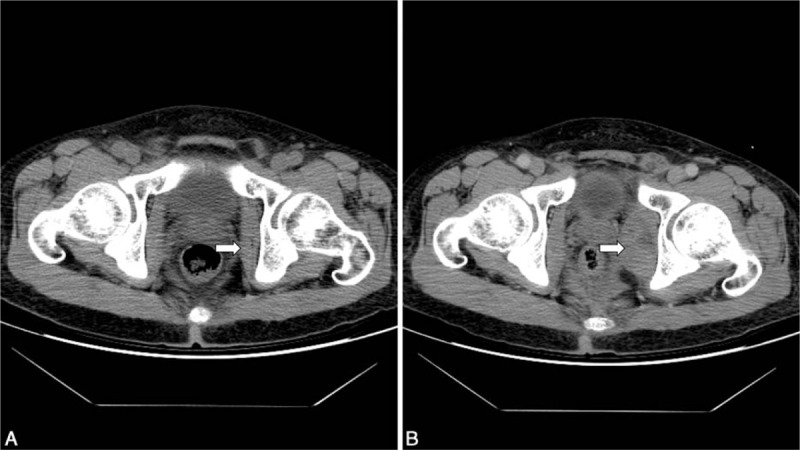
Contrast-enhanced computed tomography (CT) results for the patient: (A) CT conducted at the emergency room, indicating no specific finding of the left obturator internus muscle (arrow); and (B) CT conducted after the patient complained of buttock pain, revealing an enlarged and heterogeneously enhanced soft tissue mass in the left obturator internus muscle (arrow).
